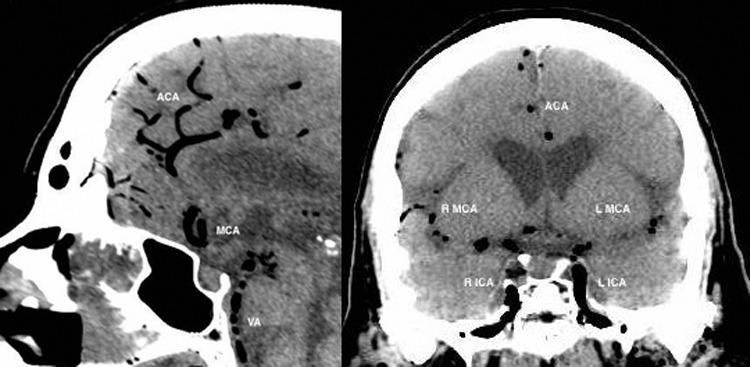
Sagittal and coronal CT head: abnormal intra-arterial gas seen within internal carotid arteries (ICA), vertebral artery (VA) and intra-cranial arteries. Air embolus is seen in bilateral peri-callosal anterior cerebral arteries (ACA) and bilateral middle cerebral arteries (MCA) (R = right; L = left).
